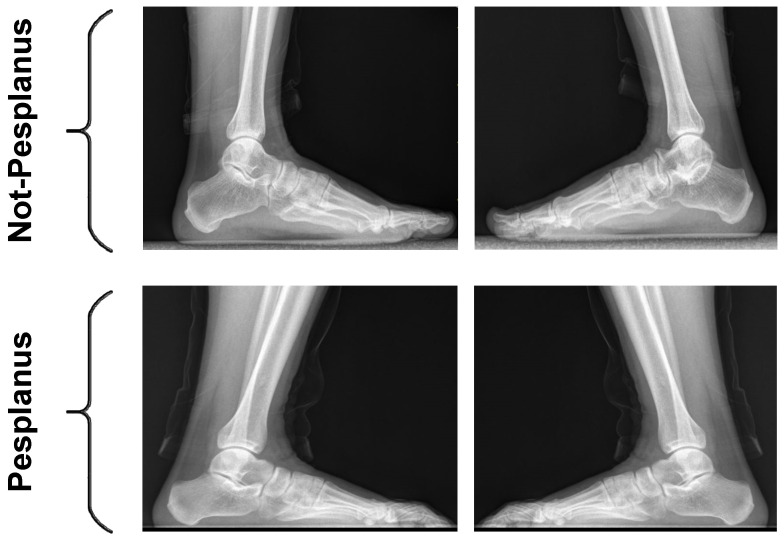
Randomly selected X-ray images from the labeled pes planus dataset.
